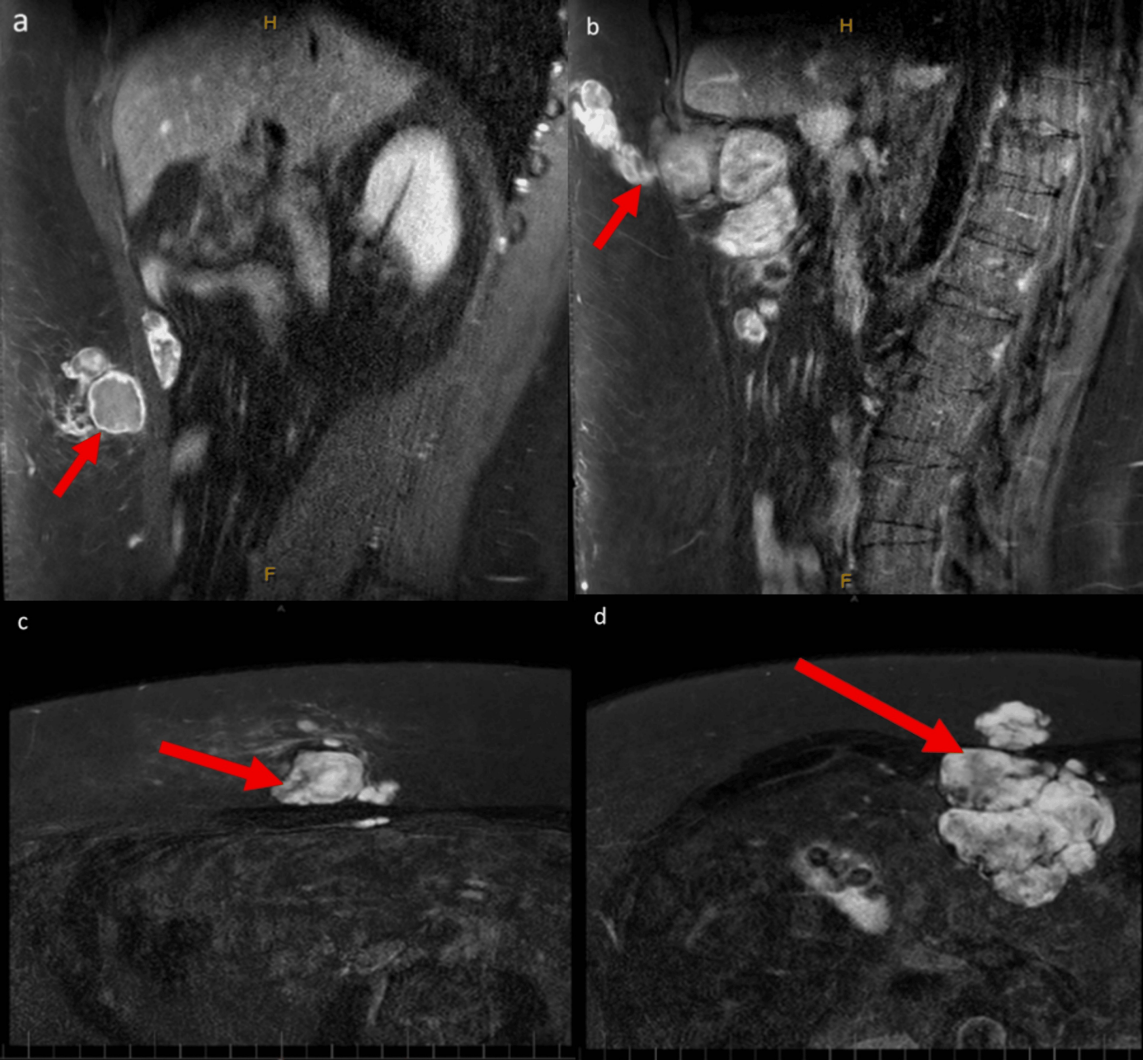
MRI T1 phase images taken pre-operatively a: Sagittal slice with 4 mm thickness, W:1977/L:1137, demonstrating the inferior abdominal wall tumour (red arrow). b: Sagittal slice with 4 mm thickness, W:1805/L:1038, demonstrating the superior abdominal wall tumour (red arrow) with intraperitoneal extension. c: Axial slice with 4 mm thickness, W:4095/L:2048, demonstrating the inferior abdominal wall tumour (red arrow). d: Axial slice with 4 mm thickness, W:4095/L:2048, demonstrating the superior abdominal wall tumour (red arrow) with intraperitoneal extension.
